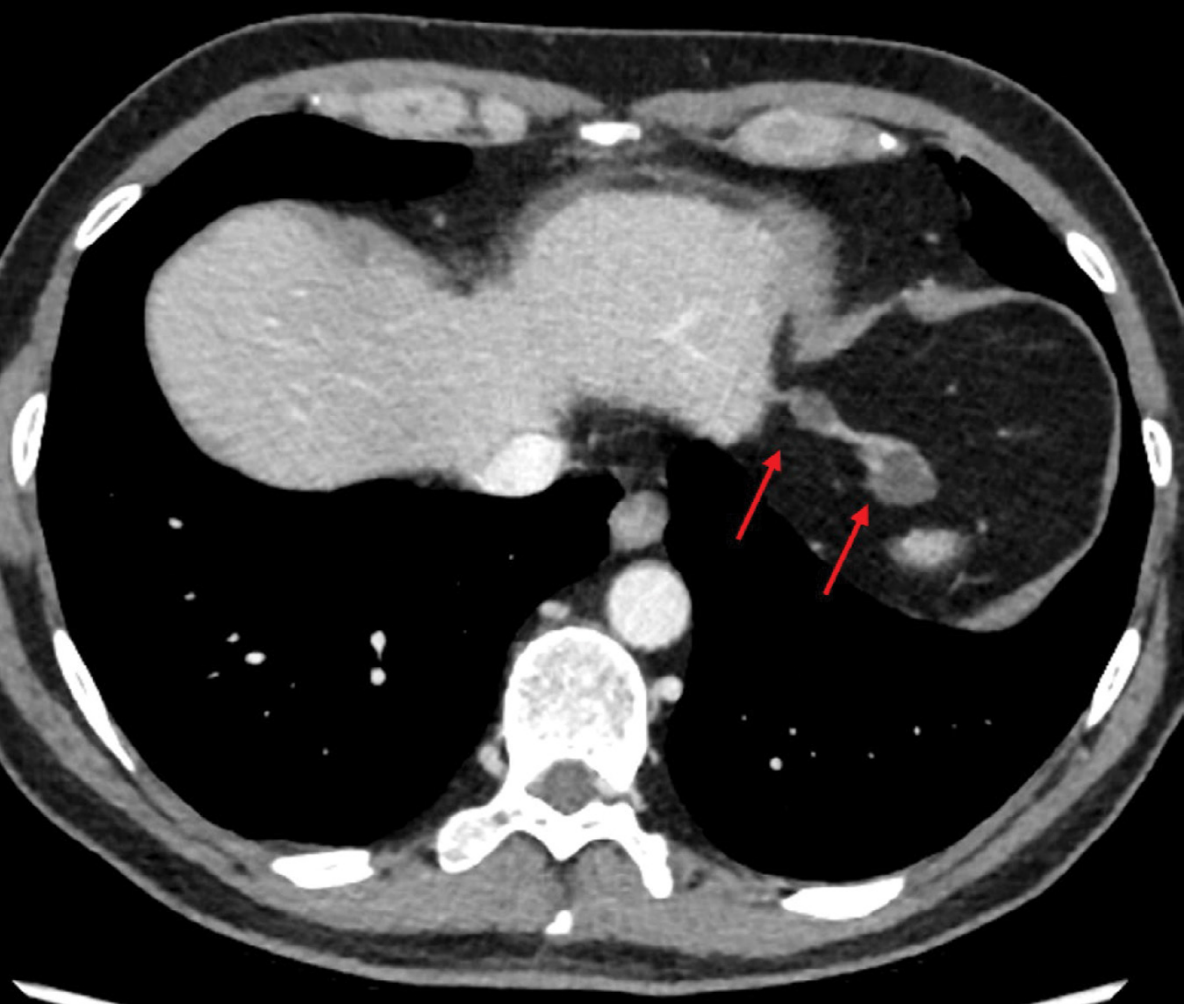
Axial view of the contrast enhanced abdominal CT‑scan showing the cystic lesions (arrows).
